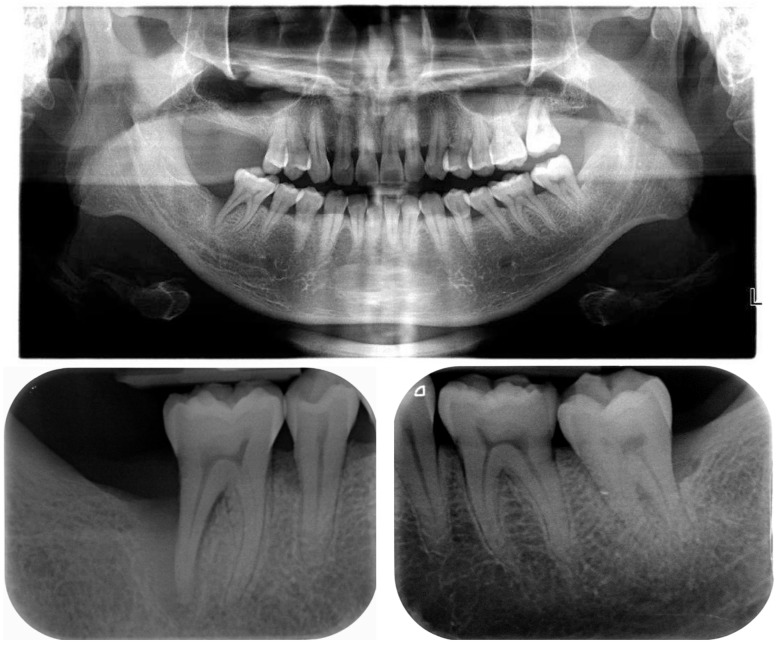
Case 1—Baseline radiographs. OPG taken in August 2018 at primary care, periapicals taken in October 2018.
